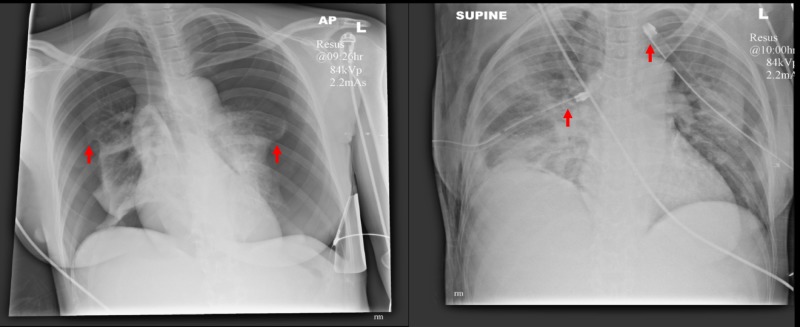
Anteroposterior chest radiographs pre- and post-drain insertion with lung edges and drain tips highlighted.
